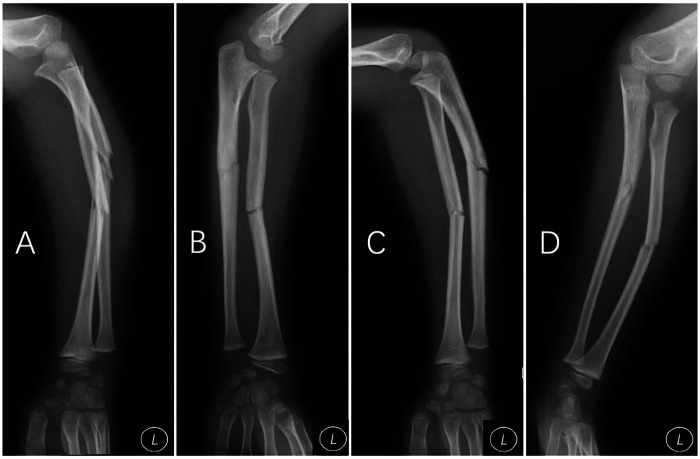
A 4-year-old male's primary forearm fracture (x-rays A, B) and refracture (x-rays C, D) occurred at the same site, as confirmed by anteroposterior and lateral comparisons.
